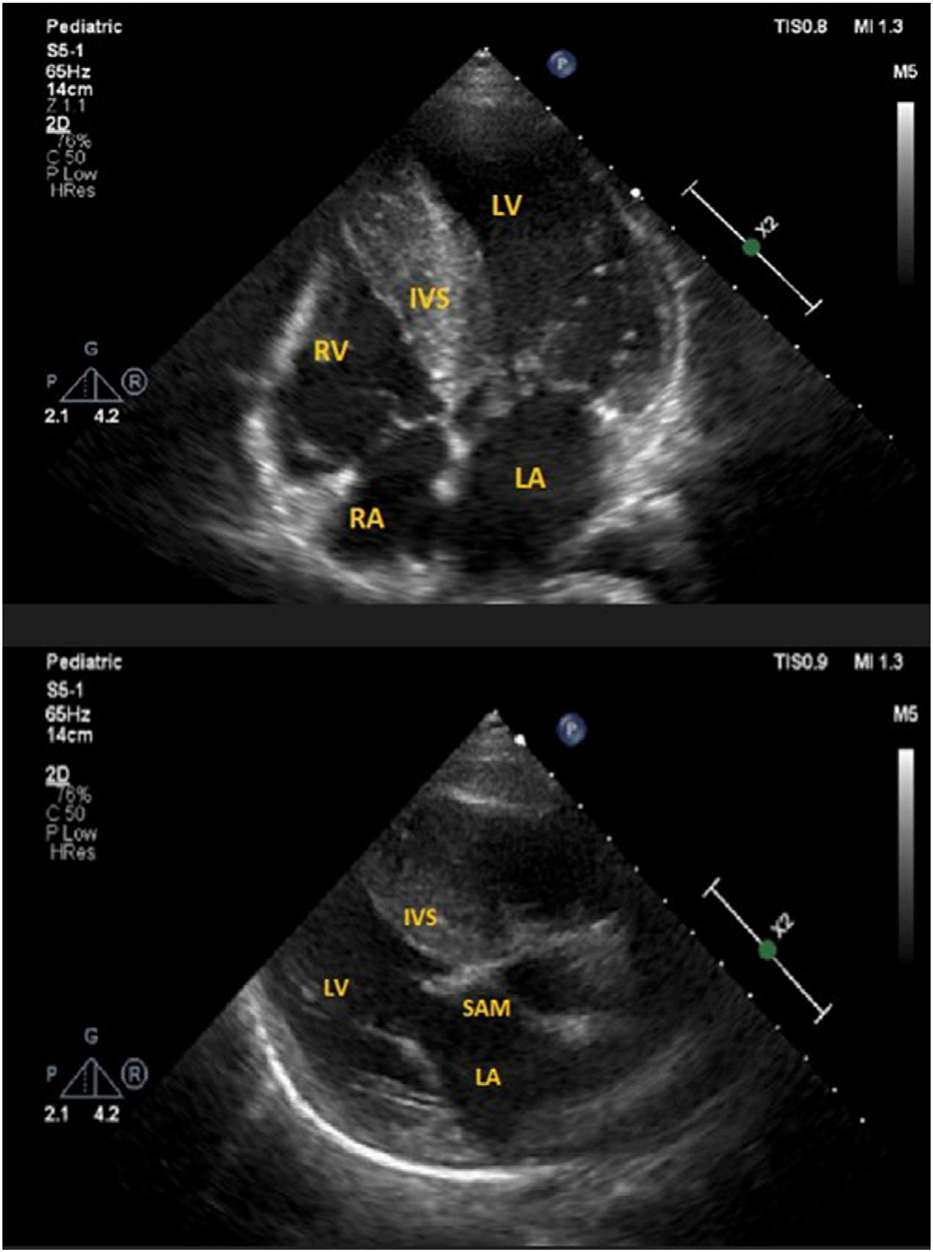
(A) ECHO 2-D showing severe interventricular hypertrophy in apical-4 chamber view in the proband. LV, left ventricle; LA, left atrium; RV, right ventricle; RA, right atrium; IVS, intraventricular septum. (B) ECHO 2-D showing systolic anterior motion (SAM) of mitral valve in parasternal long axis view in the proband. LV, left ventricle; LA, left atrium; IVS, intraventricular septum.
